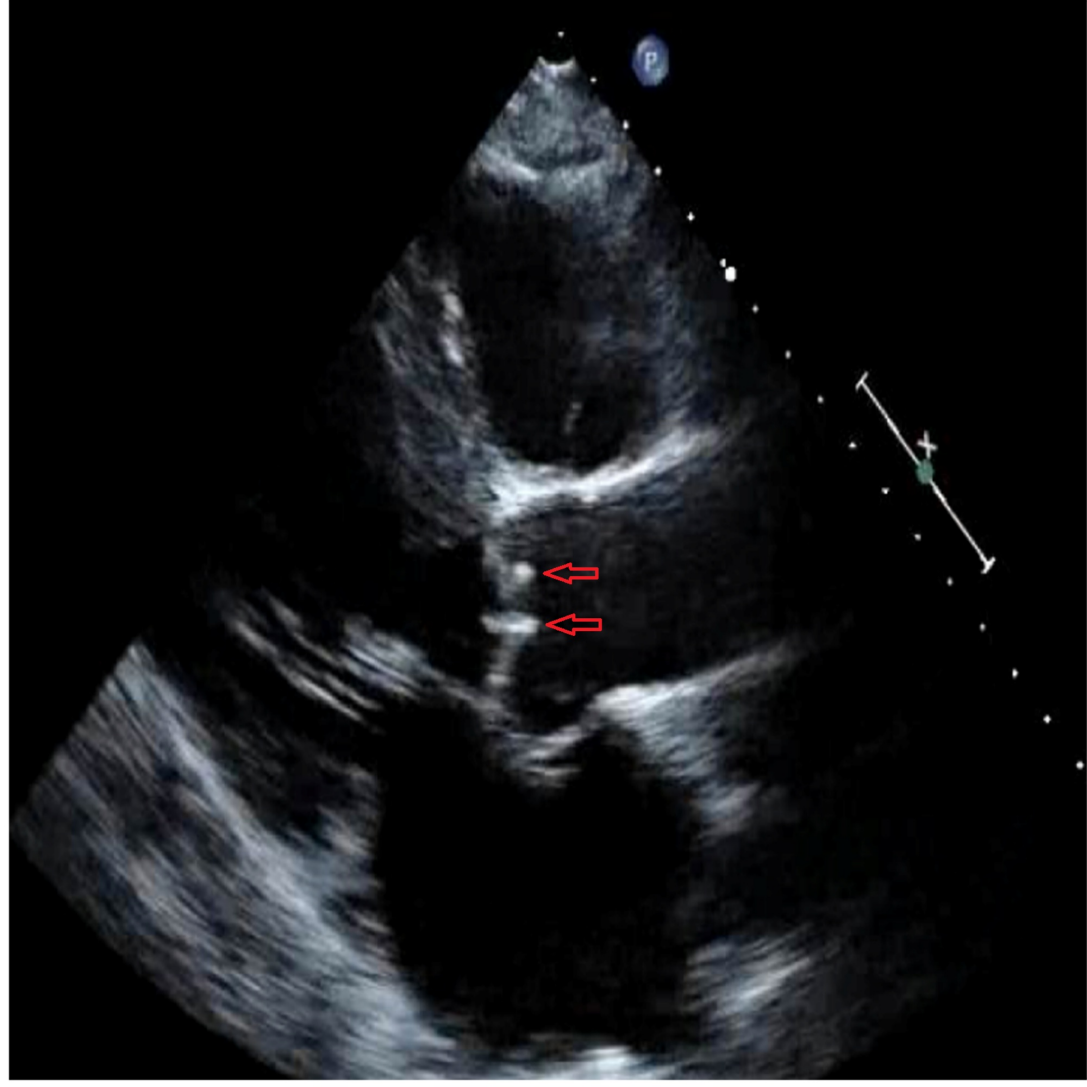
Transthoracic echocardiogram showing vegetations on aortic valve.
